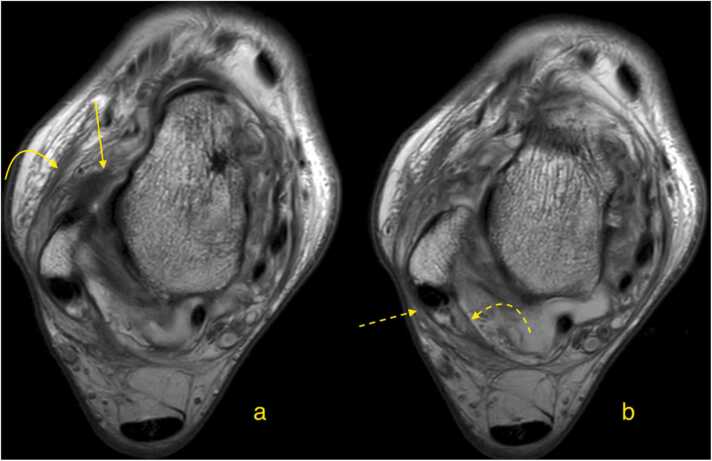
Patient after a sprain injury to the ankle. Magnetic resonance imaging (a and b) proton density-weighted, axial cross sections. The imaging revealed a complete rupture of the anterior talofibular ligament (straight arrow). Thickening of the crural fascia at the level of the lower part of the extensor retinaculum (curved arrow) is observed, along with thickening of the crural fascia at the level of the posterior boundary of the peroneal tunnel.
